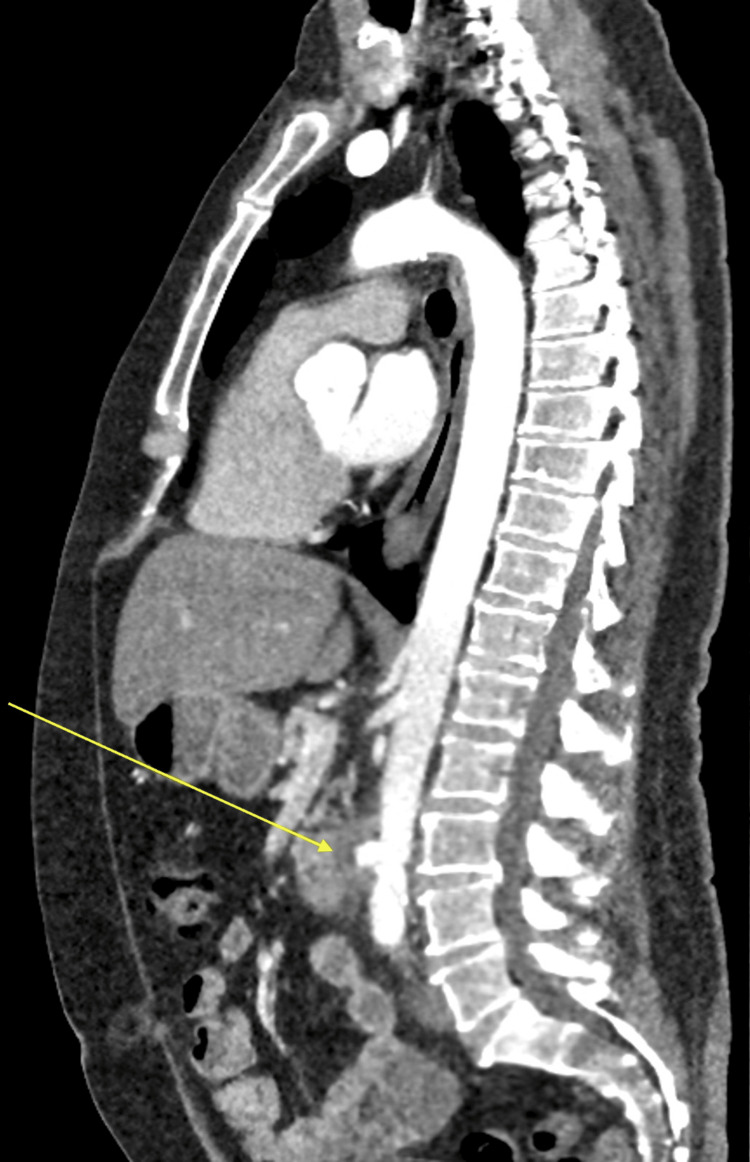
Sagittal contrast-enhanced CT of the abdomen and pelvis demonstrating progression of periaortic inflammation and anterior wall bulging of the mid-abdominal aorta, consistent with pseudoaneurysm formation.
